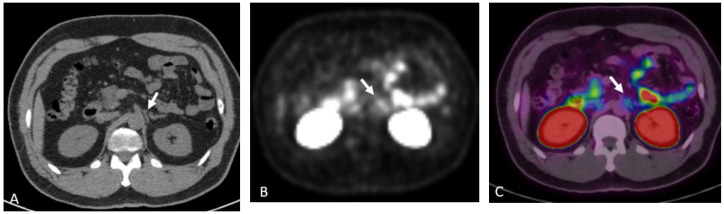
NECT (A), 18F DCFPyL PET (B), and fused PET/CT (C) images show curvilinear soft tissue, which demonstrate low-grade uptake (arrows). The location is characteristic for the celiac ganglion, a normal anatomic structure that can be mistaken for a pathologic lymph node.
